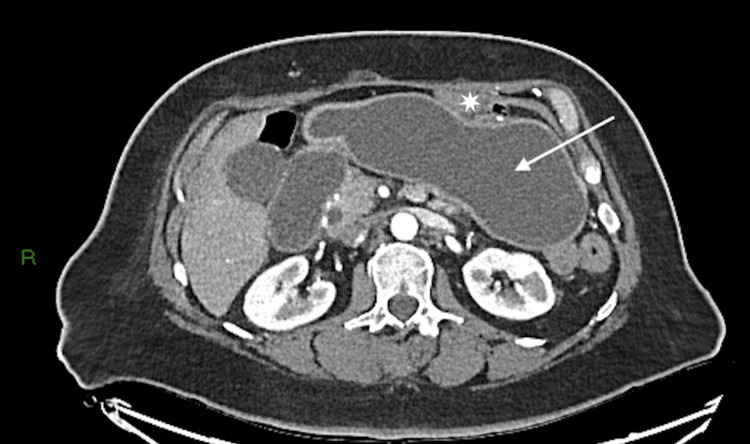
Pre-procedural axial CT demonstrating gastric remnant anatomy Axial contrast-enhanced CT image showing the relationship of the distended gastric remnant (white arrow) and the Roux limb (asterisk) to the anterior abdominal wall.
